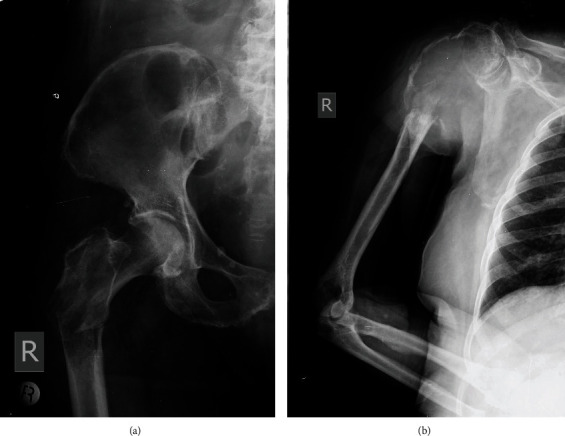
Plain radiographs ((a) anteroposterior of the right hip, (b) anteroposterior of the right shoulder and humerus) at 14 months postoperatively showing the pathological peritrochanteric and proximal humerus fractures the patient sustained following a fall.
